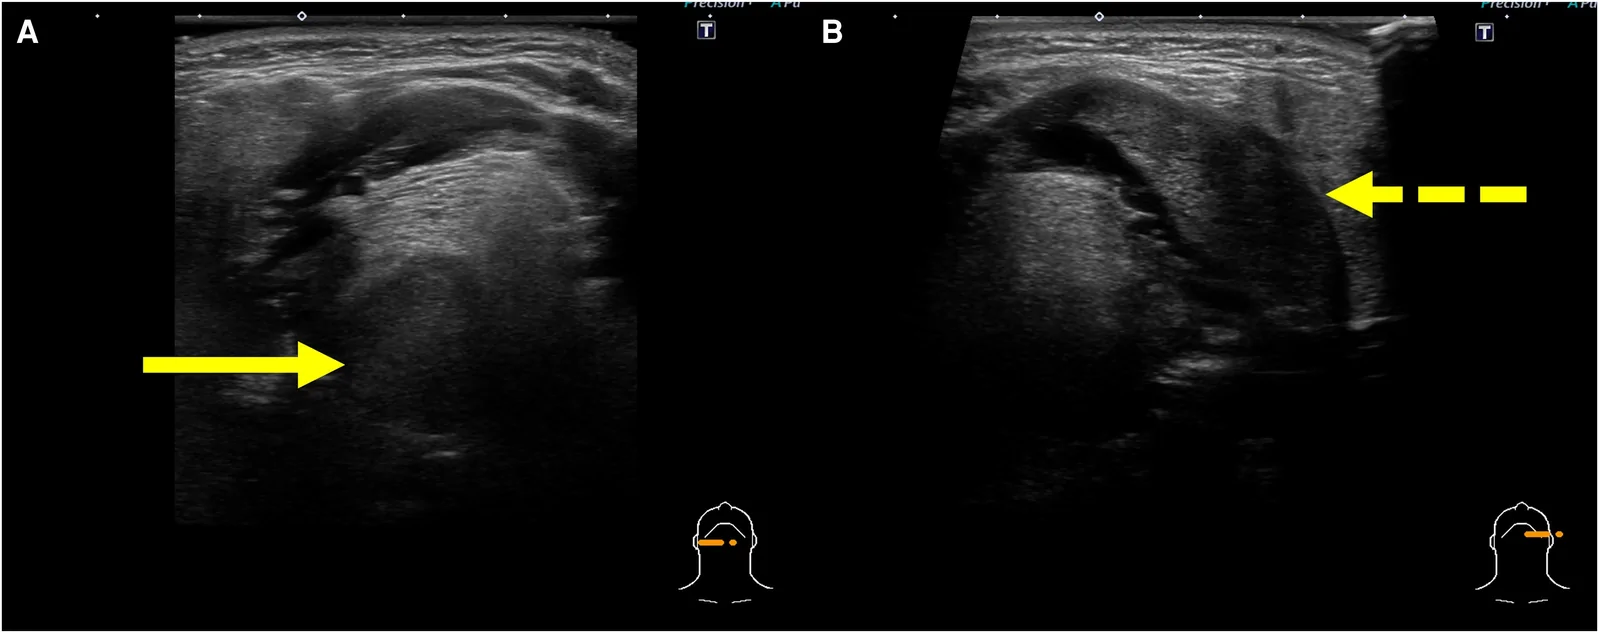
Images of thyroid ultrasonography. Two masses with clear demarcation and homogeneous internal composition. (A) At the base of the tongue. (B) At the left parapharyngeal space.
